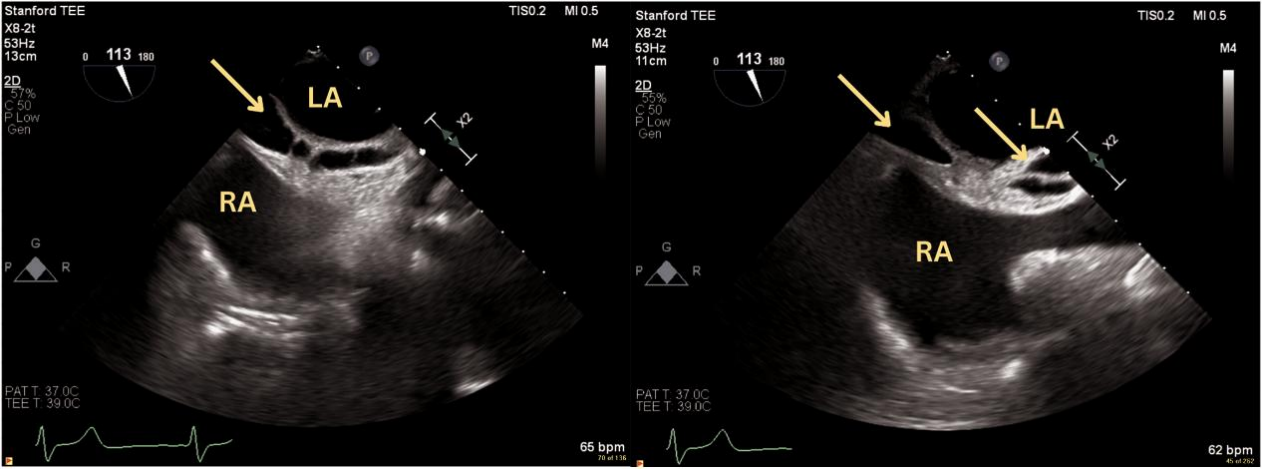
Following initial mitral valve transcatheter edge-to-edge repair device placement, a small, organizing effusion was noted within the interatrial septum, extending inferior to superior (arrows) as seen in the transoesophageal echocardiogram bi-caval view. LA, left atrim; RA, right atrium.
